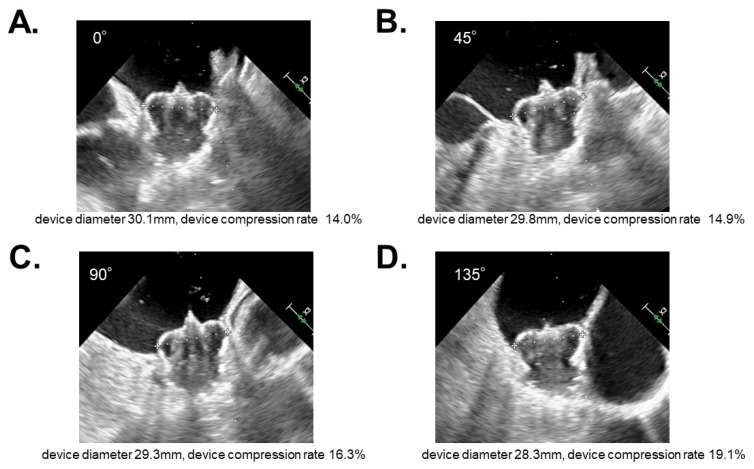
A representative case of WATCHMAN 2.5 (device size 33 mm) showing a variety of device compression rates observed by transesophageal echocardiography at 0 degrees (A), 45 degrees (B), 90 degrees (C), and 135 degrees (D).
